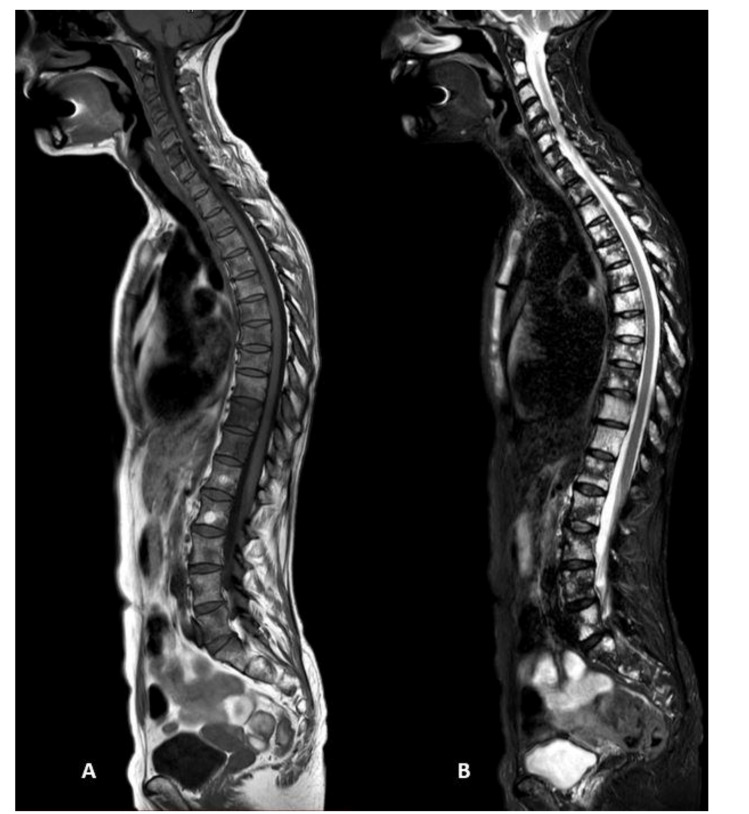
(A) T1-weighted turbo spin-echo and (B) T2-weighted short-tau inversion recovery (STIR) sequences of the spine acquired on the sagittal plane, showing multiple focal bone lesions hypointense on T1 sequences and hyperintense on T2 sequences, in a patient with focal infiltration pattern of multiple myeloma.
